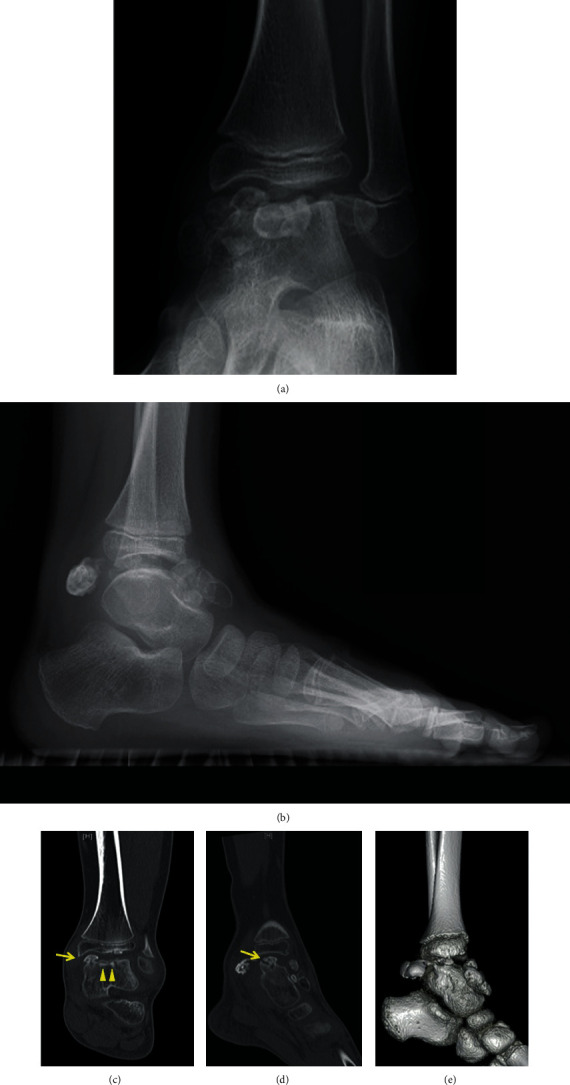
Plain radiograph: (a) anteroposterior image and (b) lateral image. Computed tomography (CT): (c) axial image, (d) sagittal image, and (e) 3-dimensional image. The left ankle plain radiograph showed multiple oval free bodies at the anterior and posterior ankle joint space. CT image showed intra-articular multiple ossified oval mass lesions (yellow arrowhead), and some protrusions arose from the talus (yellow arrow).
